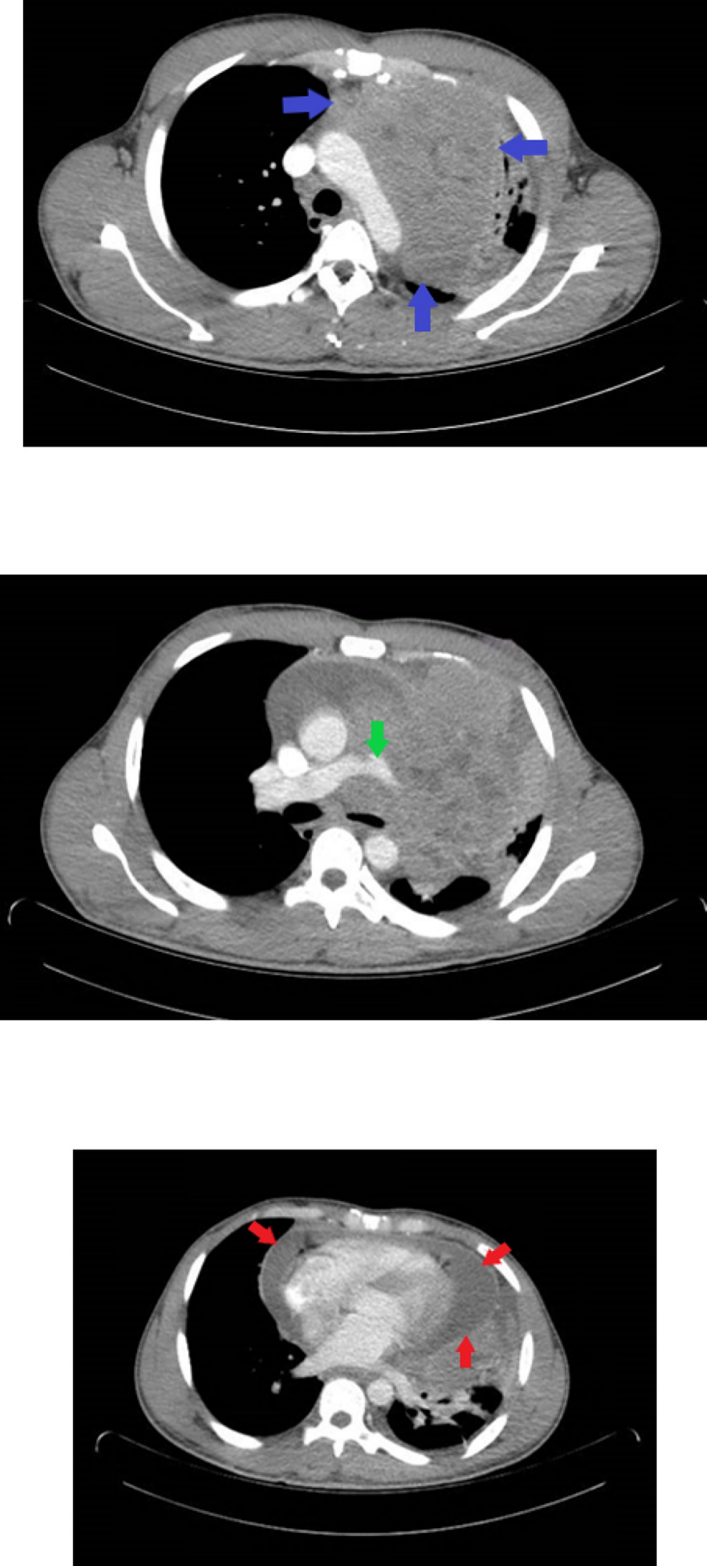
Contrast-enhanced CT of the chest. Left thoracic mass measuring 14.1 cm longitudinally, nearly 13 cm anteroposteriorly and nearly 13 cm transversely with regions of inner necrosis arising from the middle mediastinum (top image, blue arrows). Severe narrowing of the left pulmonary artery (middle image, green arrow). Moderate pericardial effusion is noted as well (lower image, red arrows)
